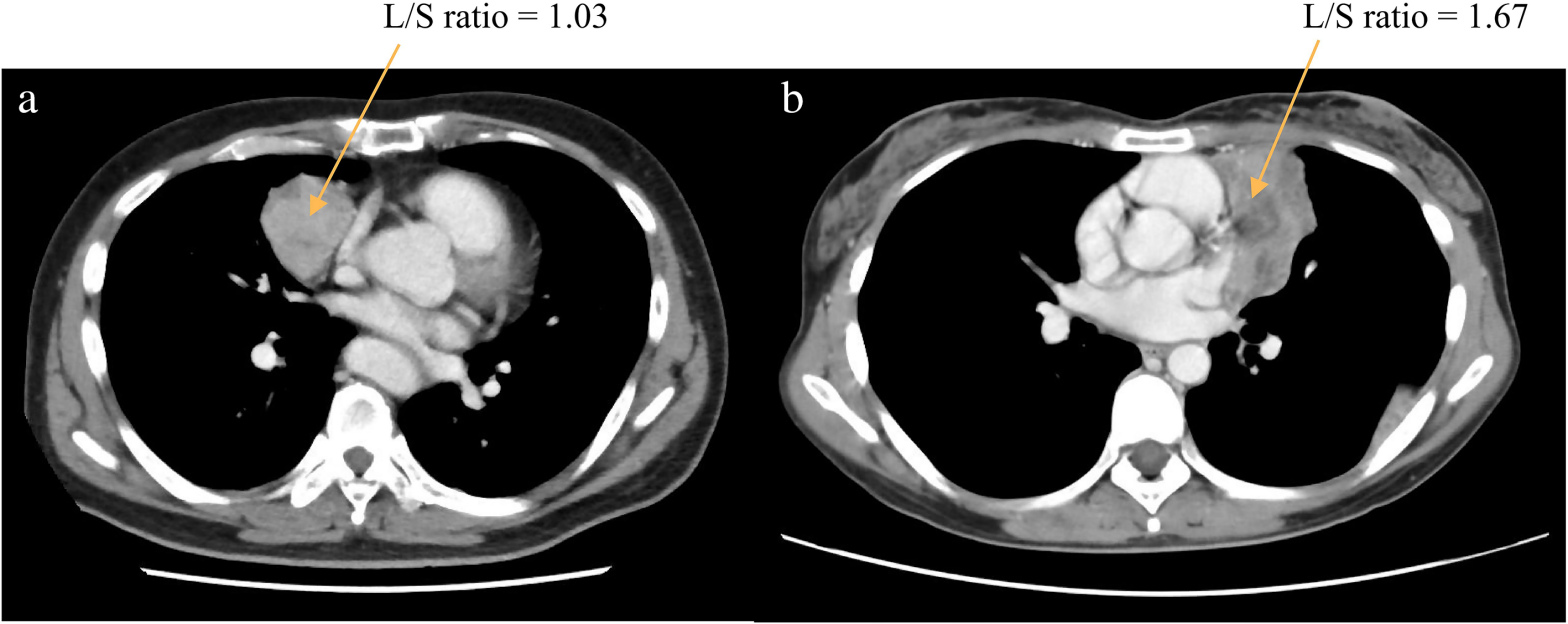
Computed tomography images of two representative patients for L/S ratio. An example is a 61‐year‐old man with a low L/S ratio (1.03) thymoma (maximum diameter = 8 cm; minimum diameter = 7.8 cm) and TNM stage I who was still alive at the last follow‐up (a). Another example is a 34‐year‐old woman with a high L/S ratio (1.67) thymoma (maximum diameter = 12.5 cm; minimum diameter = 7.5 cm) and TNM stage II who was dead at the last follow‐up (b). L/S, long‐to‐short axis
